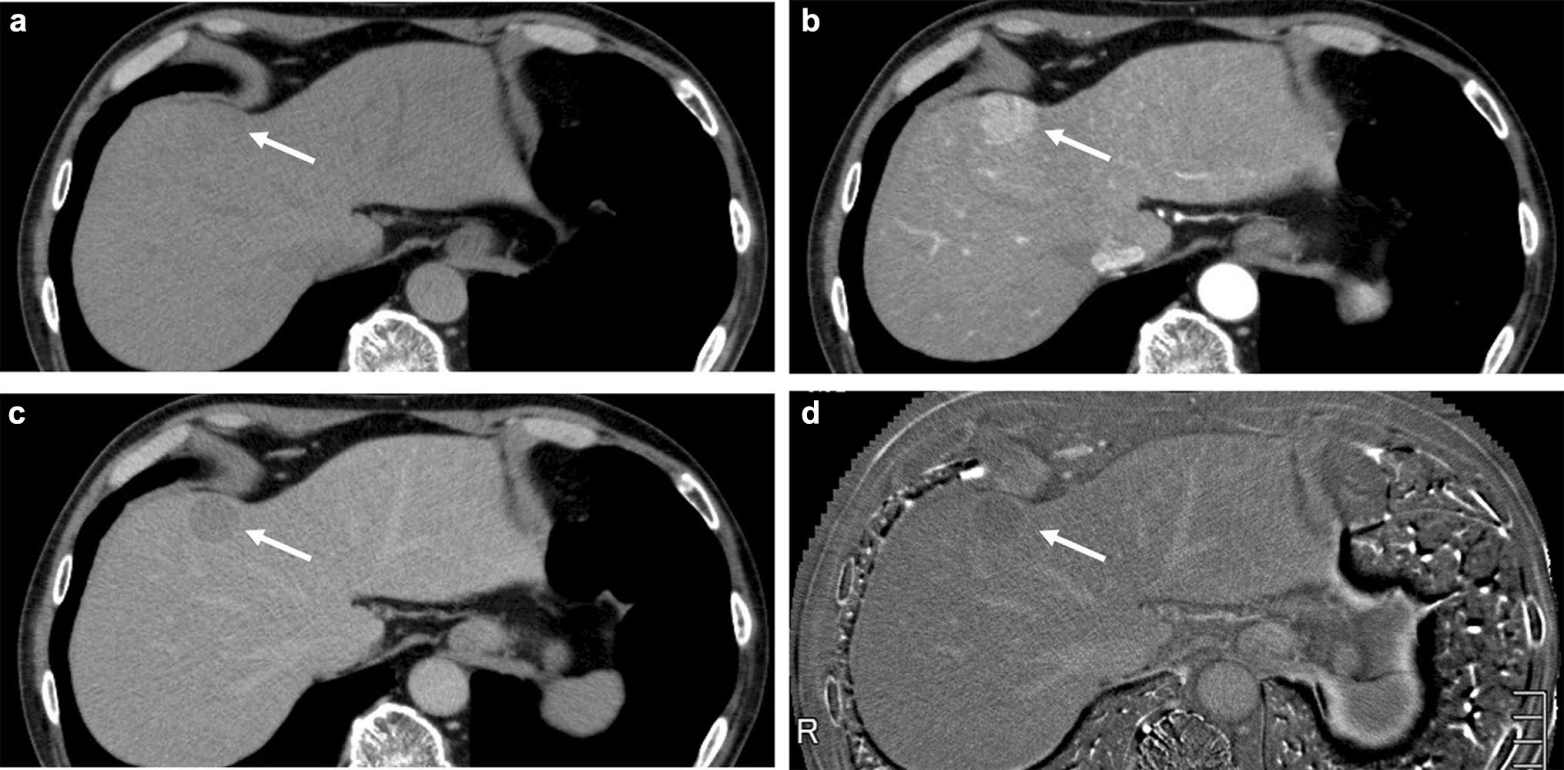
63 year-old man with chronic hepatitis C infection. Pathologically, a well differentiated hepatocellular carcinoma, mainly consisting of trabecular component, associated with fibrous capsule, was diagnosed. No septal formation or fatty change was evident. a Precontrast CT. The tumor exhibits almost iso- to minimally low density (arrow). Measured density were 55 and 59 Hounsfield units for the tumor and the background liver, respectively. b Arterial phase CT. The tumor shows homogeneous enhancement (arrow). c Equilibrium phase CT. The tumor exhibits apparent “washout” (arrow). d Extracellular volume fraction (ECV) map reveal apparently lower value of the tumor, as compared to the background liver. Measured ECV were 20.2 and 26.1% for the tumor and the background liver, respectively. In this case, although the precontrast density of the tumor or the liver were almost identical, it was considered apparently lower ECV of the tumor contributed to the positive washout
